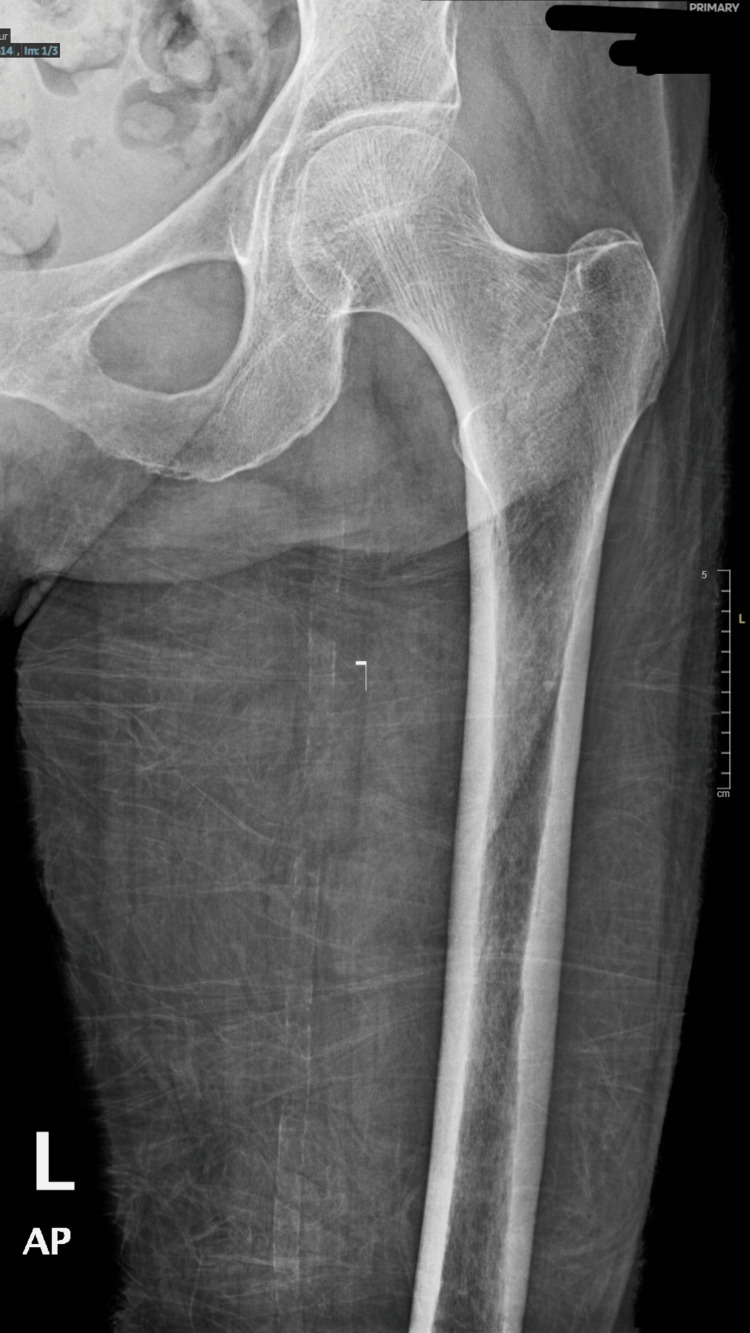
Plain radiograph shows no definite fracture lines or osseous lesions
